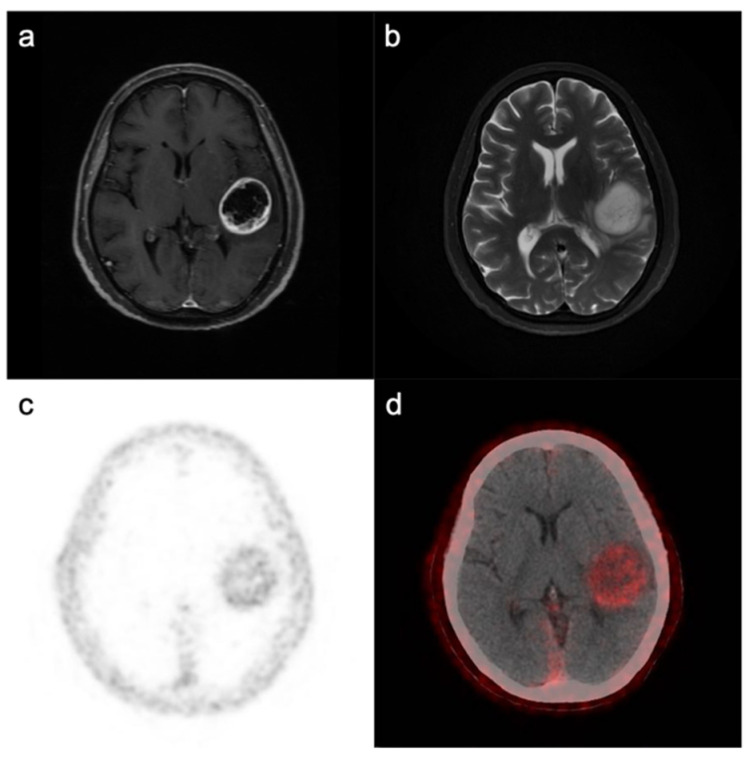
Magnetic resonance imaging (MRI) and FAPI PET/CT in GBM. An IDH-wildtype GBM from a 71-year-old woman displayed ring-like contrast enhancement with hypointense T1-weighted and hyperintense T2-weighted signals (a,b) from central tissue. ^68^Ga-FAPI PET and merged PET/CT images (c,d) showed elevated radioactivity in the whole tumor area (including ring-like contrast enhancement and noncontrast-enhanced tissue).
